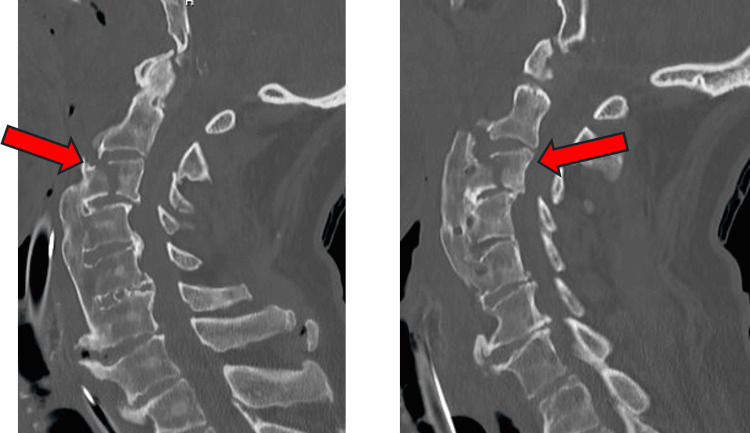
Patient 8 neck CT without contrast Arrows indicating C3 vertebral fracture
